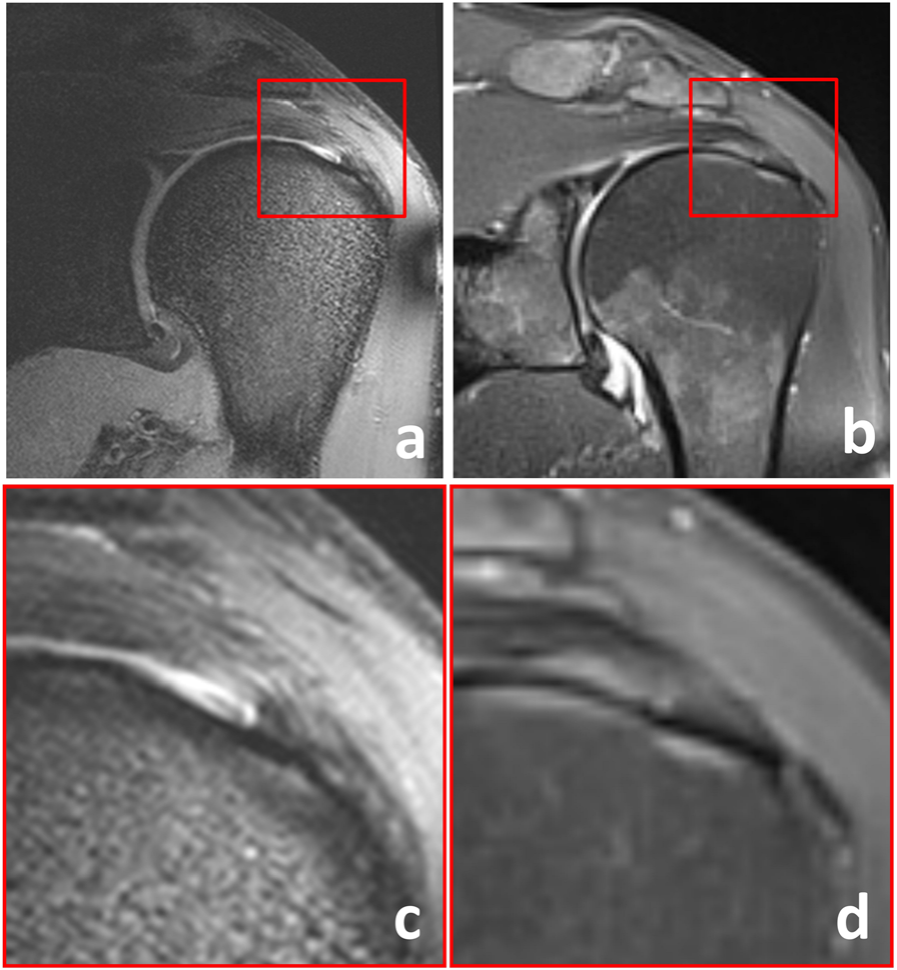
Example of overrating with 7-T MRI. In this 52-year-old male patient, a partial tear of the supraspinatus tendon was suspected according to 7-T MRI based on the signal increase and fluid collection near to the footprint (a, c). At 1.5 T (b, d), these changes are less impressive. Arthroscopy revealed little scar tissue and slight thinning of the tendon, but no tendinopathy or tear. Retrospectively, the signal changes observed at 7 T must be assigned to B_1_^+^ inhomogeneities and/or the magic angle effect
